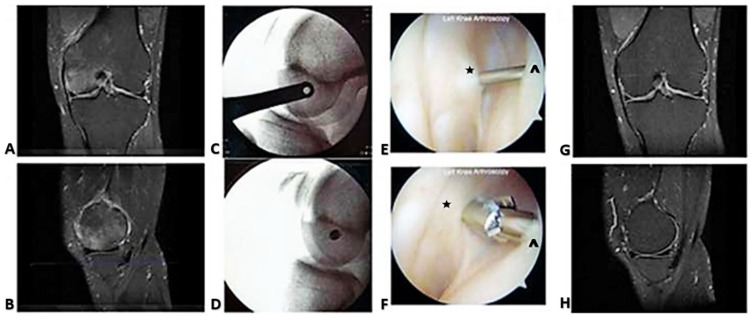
Surgical decompression of BME. (A) Coronal and (B) sagittal sections display the preoperative high signal intensity in the medial femoral condyle on the MRI T2 FS sequence. (C) Lateral X-ray views show an example of fluoroscopic guidance of pin placement, with the pin appearing as a dot (D), indicating parallelity with the fluoroscopic beam. (E, F) Arthroscopic guidance of pin entry and 4 mm cannulated drill tip through the joint capsule (*) to cancellous bone (^). (G) Coronal and (H) lateral MRI sections show resolution of the high signal intensity 3 months after decompression.
